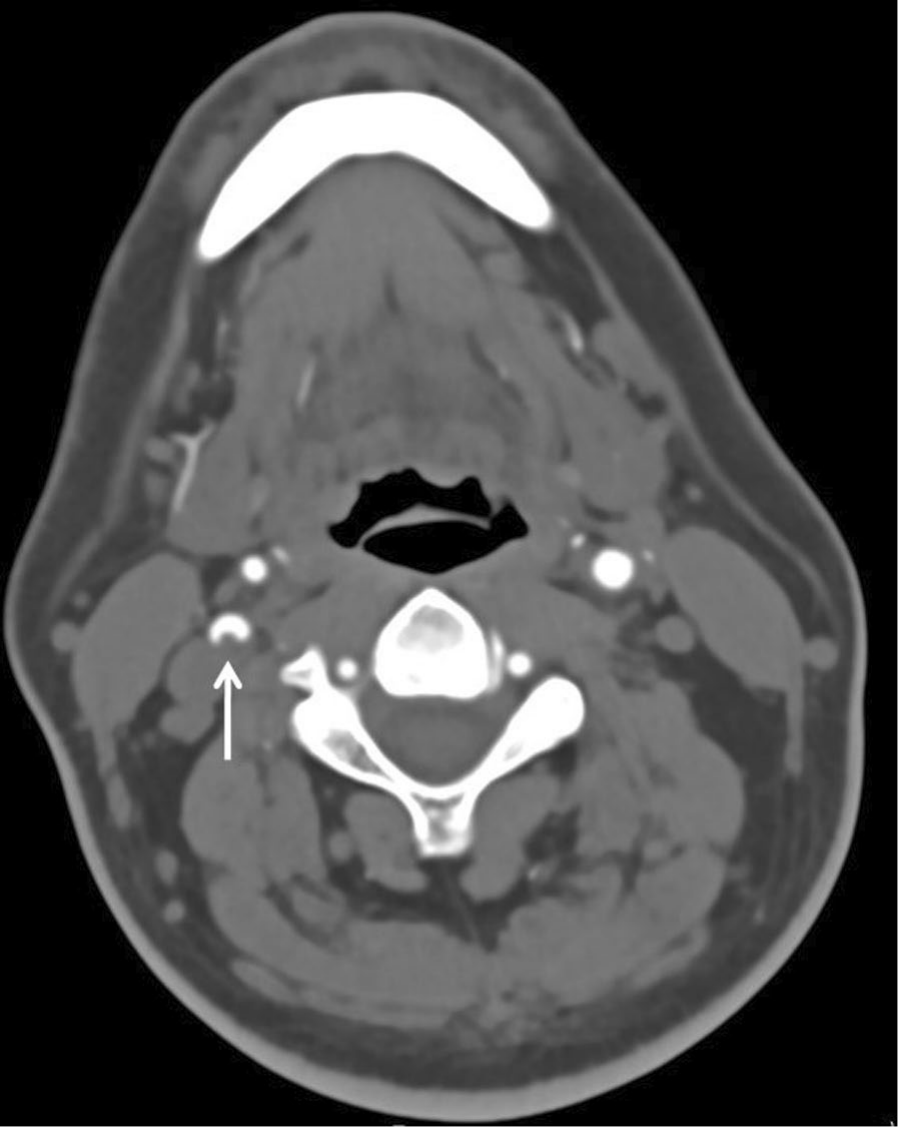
Internal carotid artery thrombus. 36-year-old-man with COVID-19 transferred to ICU on extracorporeal membrane oxygenation, developed acute onset of quadriplegia. Axial image from a CT angiogram shows acute thrombus within the right internal carotid artery (arrow). Atherosclerotic changes were not present in the remainder of the intra- and extracranial arterial vasculature
